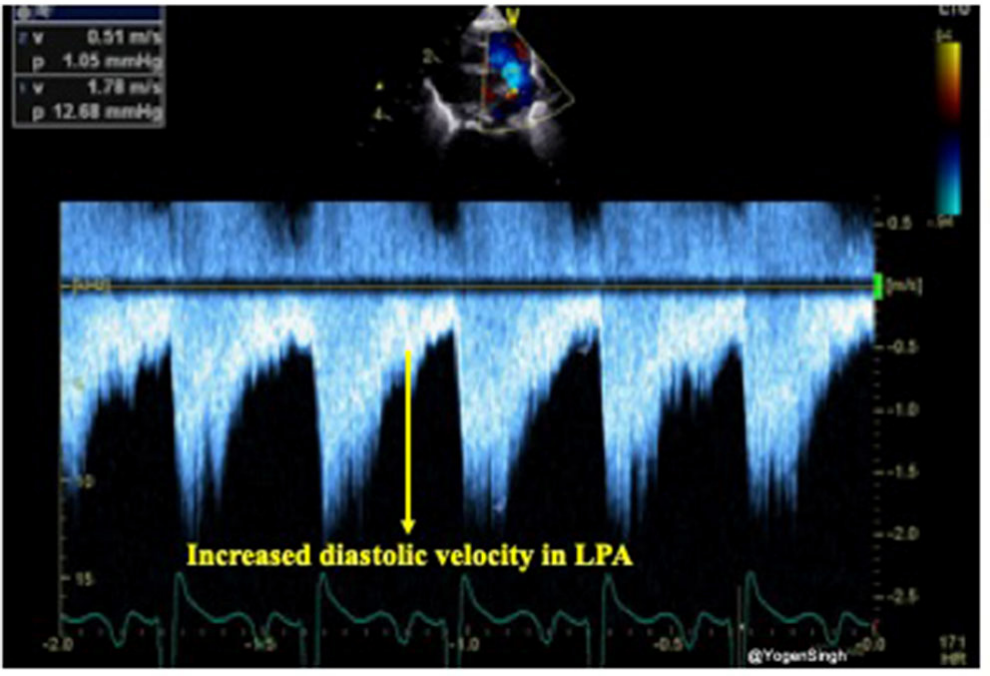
Doppler assessment of blood flow in left pulmonary artery (LPA) showing increased diastolic velocity indicative of significant ductal shunt in diastole leading to turbulence and increased velocity.
